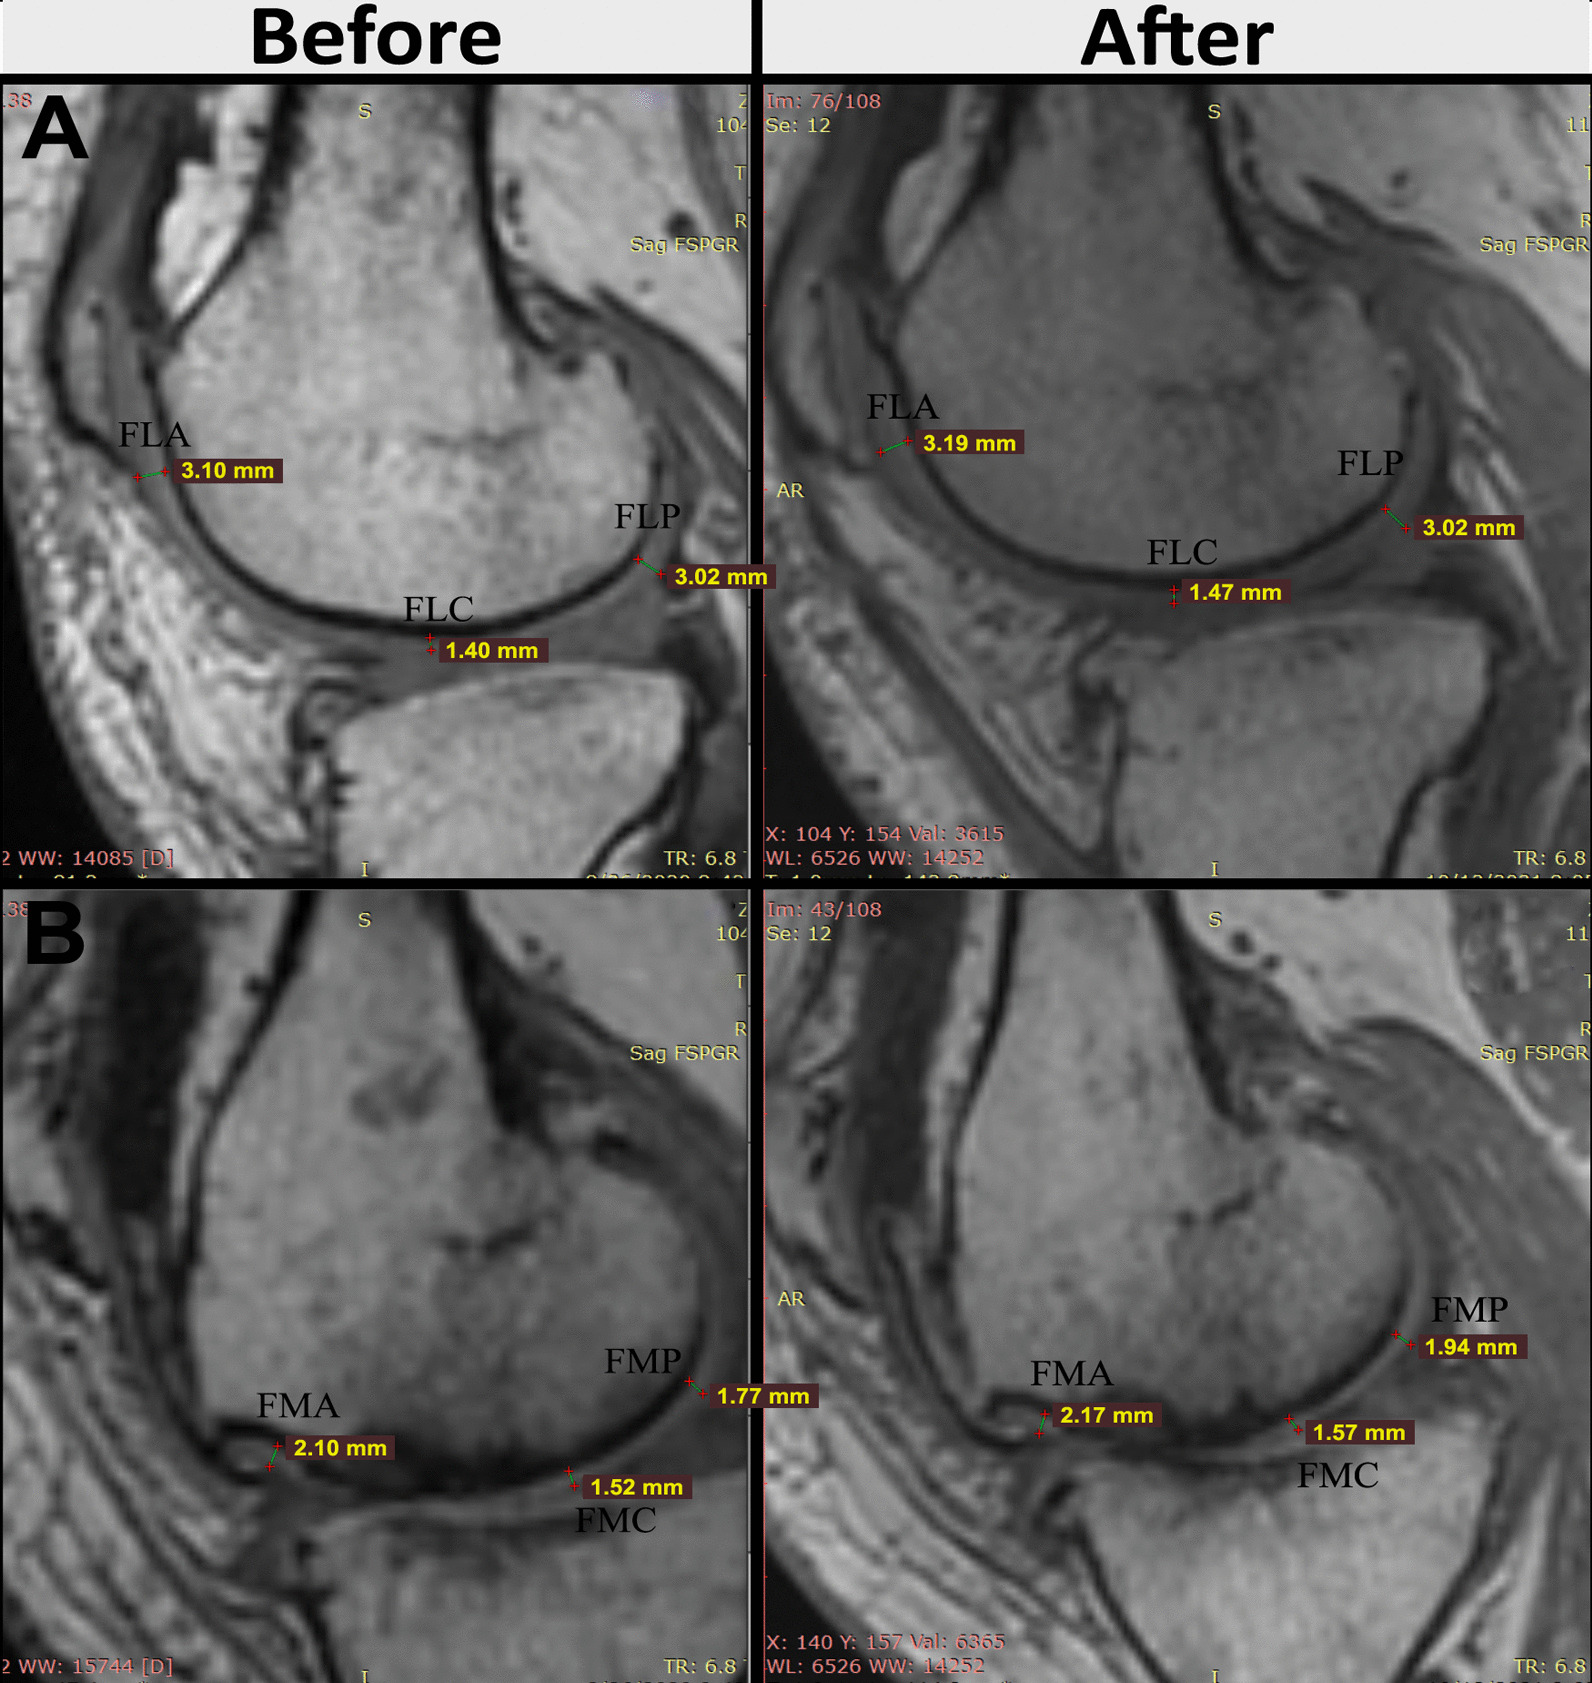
MRI analysis of femural condyle cartilage before and 48 weeks after AD-MSCs injection. A lateral radiograph indicates enhancement in anterior, posterior, and central area of femoral condyle cartilage. B Medial radiograph revealed increase in the thickness of femoral condyle cartilage in anterior, posterior, and central area. *MRI* magnetic resonance imaging, *FLC* Femur lateral central, *FLP* femur lateral posterior, *FLA* femur lateral anterior, *FMC* femur medial central, *FMP* femur medial posterior, *FMA* femur medial anterior, *AD-MSCs* adipose-derived mesenchymal stromal cells
